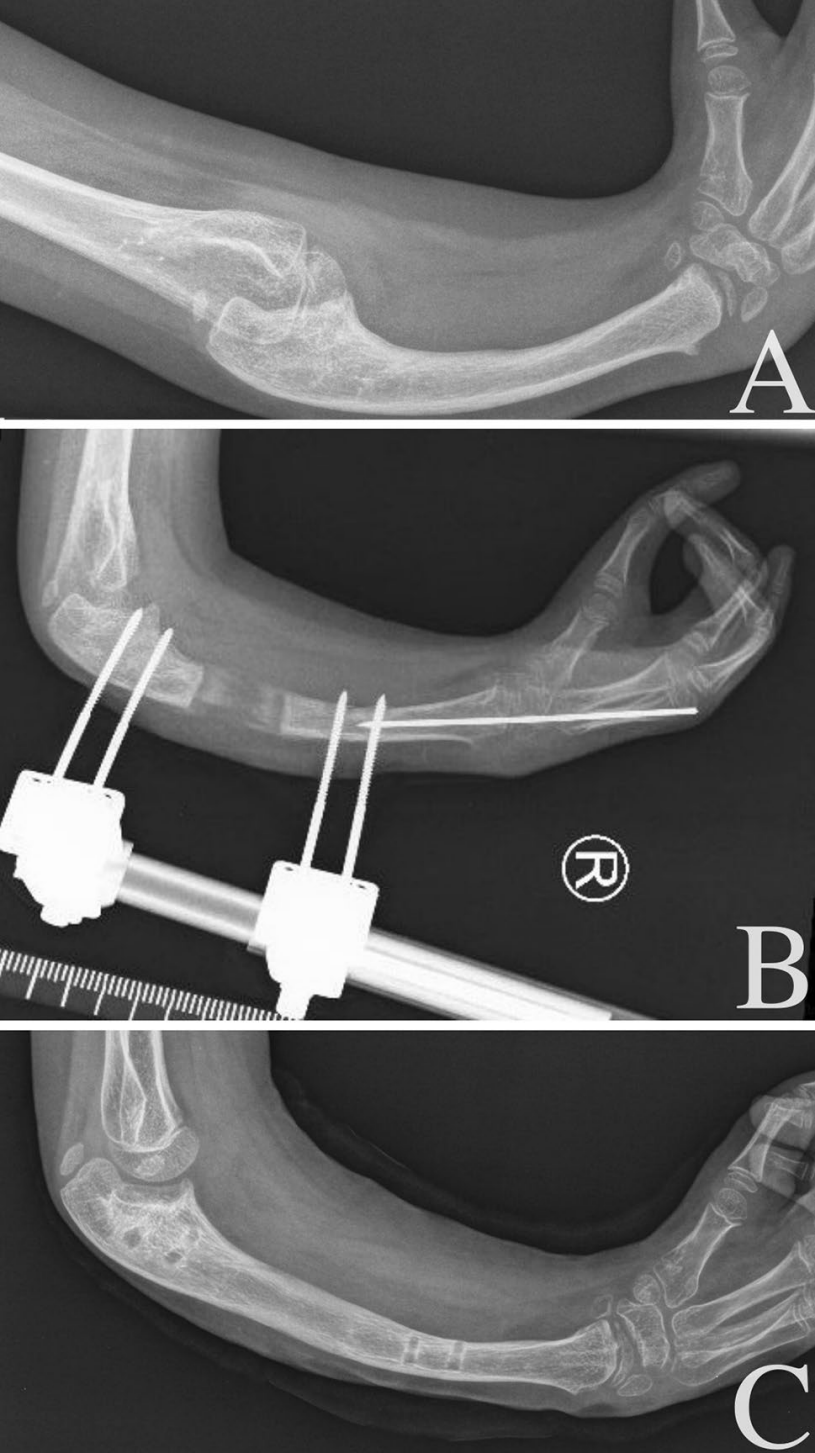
X-ray of the ulna before (a), during (b), and after (c) lengthening
